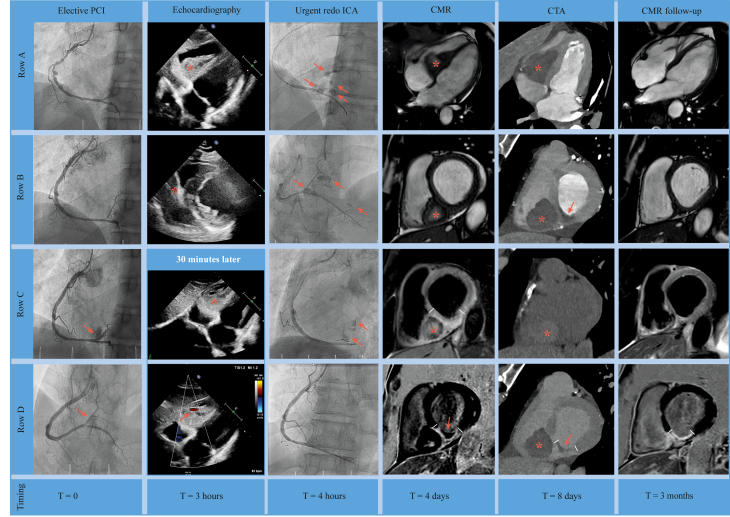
Multimodality Imaging of Intramyocardial Dissecting Hematoma The first column shows elective PCI (T = 0): row A = coronary angiography of RCA before stenting, row B = result after placement of 2 drug-eluting stents, and rows C and D = contrast extravasation at 1 site (arrow). The second column shows echocardiography (T = 3 hours): row A = subcostal view showing septal intramyocardial mass with partial occlusion of the right ventricle (asterisk), row B = parasternal short-axis view showing septal intramyocardial mass (asterisk), and rows C and D = repeated echocardiography after 30 minutes, showing subcostal view with rapid progression of intramyocardial mass (asterisk), with suggestion of flow inside this mass based on color Doppler imaging (arrow). The third column shows urgent redo ICA (T = 4 hours): rows A and B = urgent coronary angiography (2nd procedure) showing contrast extravasation at multiple sites (arrow), row C = persistent contrast extravasation despite prolonged balloon inflations (arrow), and row D = covered stent placement sealed the perforation and ended the extravasation. The fourth column shows CMR (T = 4 days): rows A and B = long-axis 4-chamber and short-axis cine images showing intramyocardial hematoma (asterisk) with partial (not obstructive) occlusion of the right ventricle, row C = short-axis T2-weighted imaging showing hyperintensity consistent with edema (between lines) and intramyocardial hematoma (asterisk); and row D = short-axis late enhancement imaging showing transmural hyperenhancement consistent with myocardial infarction inferior (between lines) and microvascular obstruction (MVO) (arrow). The fifth column shows CTA (T = 8 days): row A = long-axis 4-chamber view after intravenous contrast injection, showing large intramyocardial hematoma (asterisk) without active contrast leakage; row B = corresponding short-axis view showing large intramyocardial hematoma (asterisk) and signs of MVO (arrow), row C = native scan (without iodin contrast agent) short-axis view showing increased signal consistent with hematoma (asterisk), and row D = short-axis late enhancement imaging showing large intramyocardial hematoma (asterisk) and signs of transmural myocardial infarction (between lines) and MVO (arrow). The last column shows CMR (T = 3 months): comparable images with fourth column, showing near-complete resolution of intramyocardial hematoma (rows A and B), no more signs of edema (row C), and chronic transmural infarction (between lines) without MVO (row D). CMR = cardiac magnetic resonance; CTA = computed tomography angiography; ICA = invasive coronary angiography; PCI = percutaneous coronary intervention.
